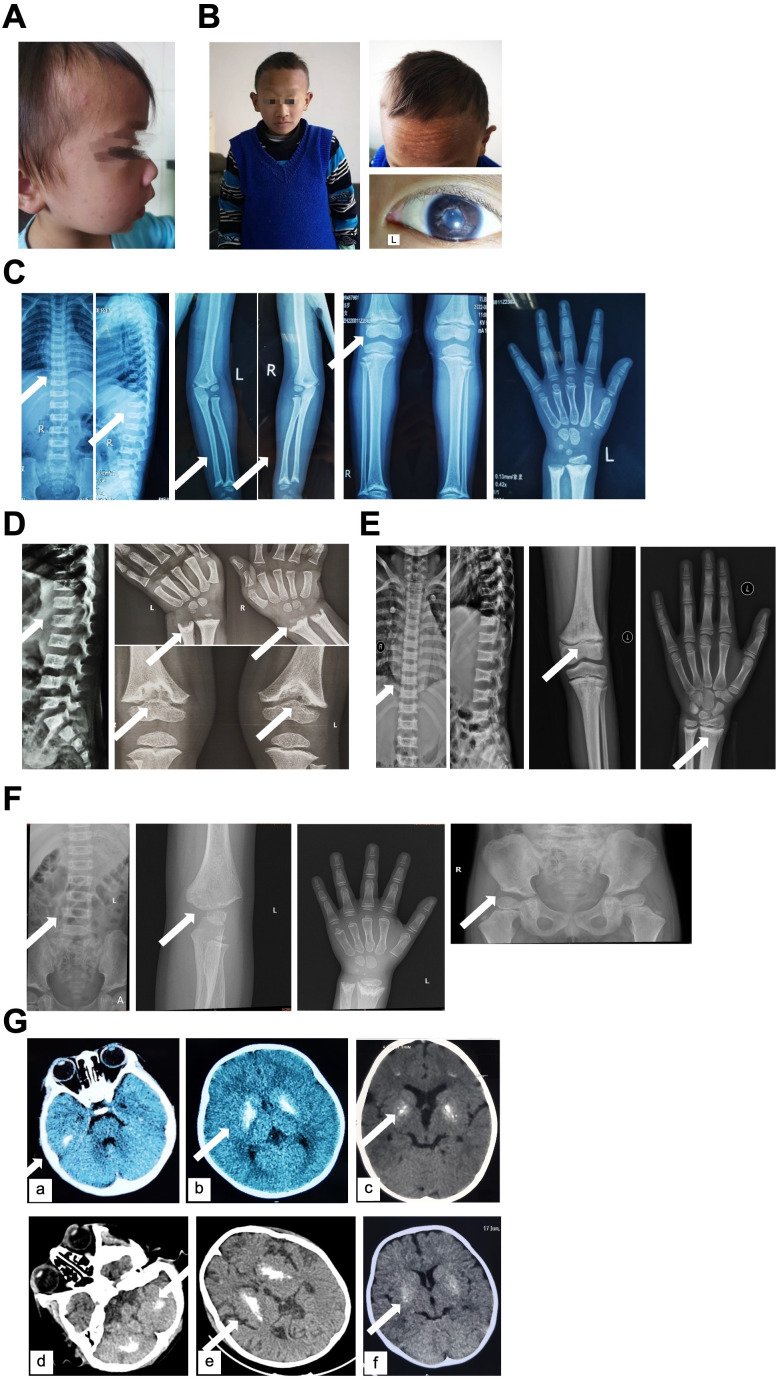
Clinical and radiological features of SPENCD patients. (A) Frontal and facial photograph of Patient 1 at the age of 5 years and 6 months. (B) Upper-body photograph of Patient 3 at 15 years of age, together with representative images showing frontal facial hyperpigmented lesions and uveitis of the eye. (C–F) Representative radiographic and computed tomography (CT) images from Patient 1 (C), Patient 2 (D), Patient 3 (E), and Patient 4 (F). Arrows indicate areas of abnormal bone structure or bone destruction. (G) Cranial CT images showing intracranial abnormalities in Patient 1 (a, b), Patient 2 (c), Patient 3 (d, e), and Patient 4 (f).
